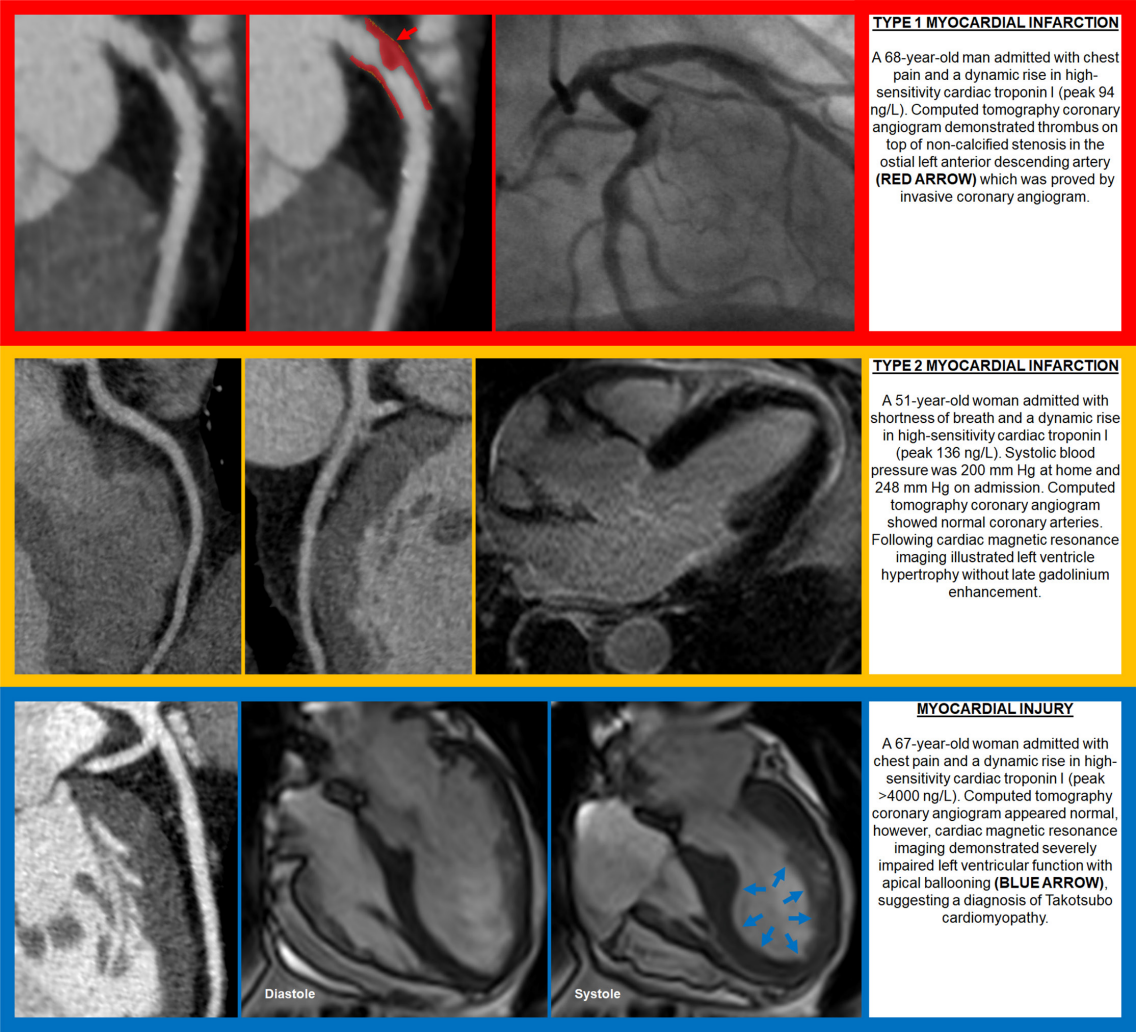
Examples showing the use of computed tomography coronary angiography in differentiation between aetiologies for cardiac troponin elevation
